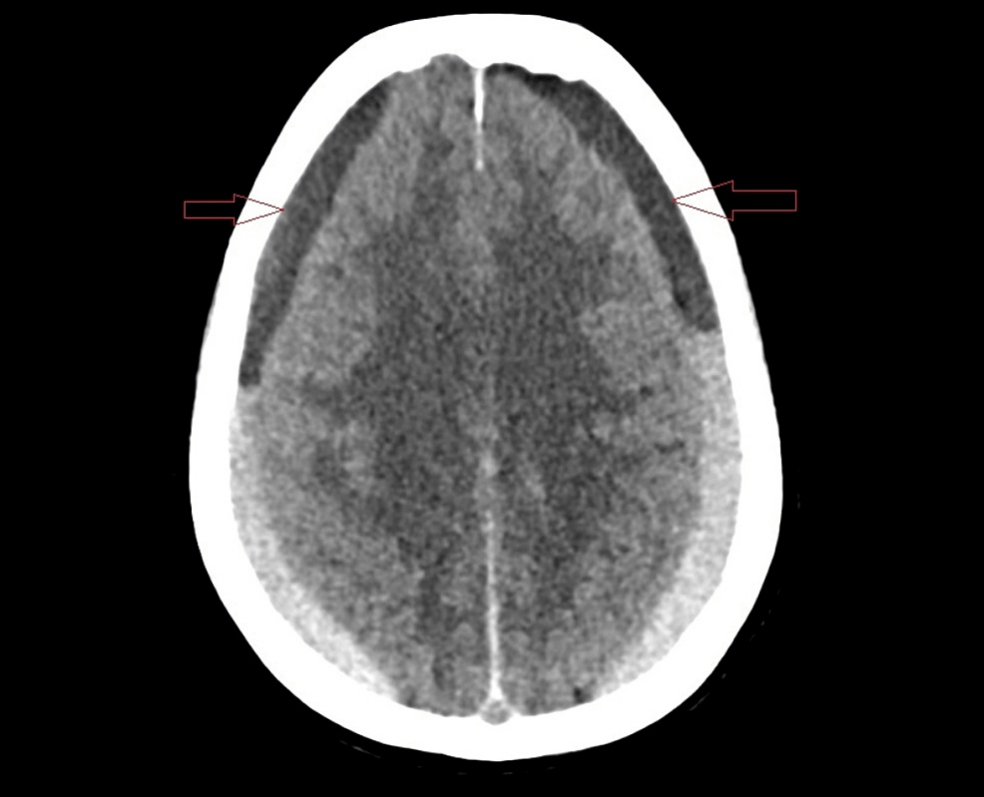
Computed tomography of the head during her second presentation showing signs of subdural hematoma (red arrows)
